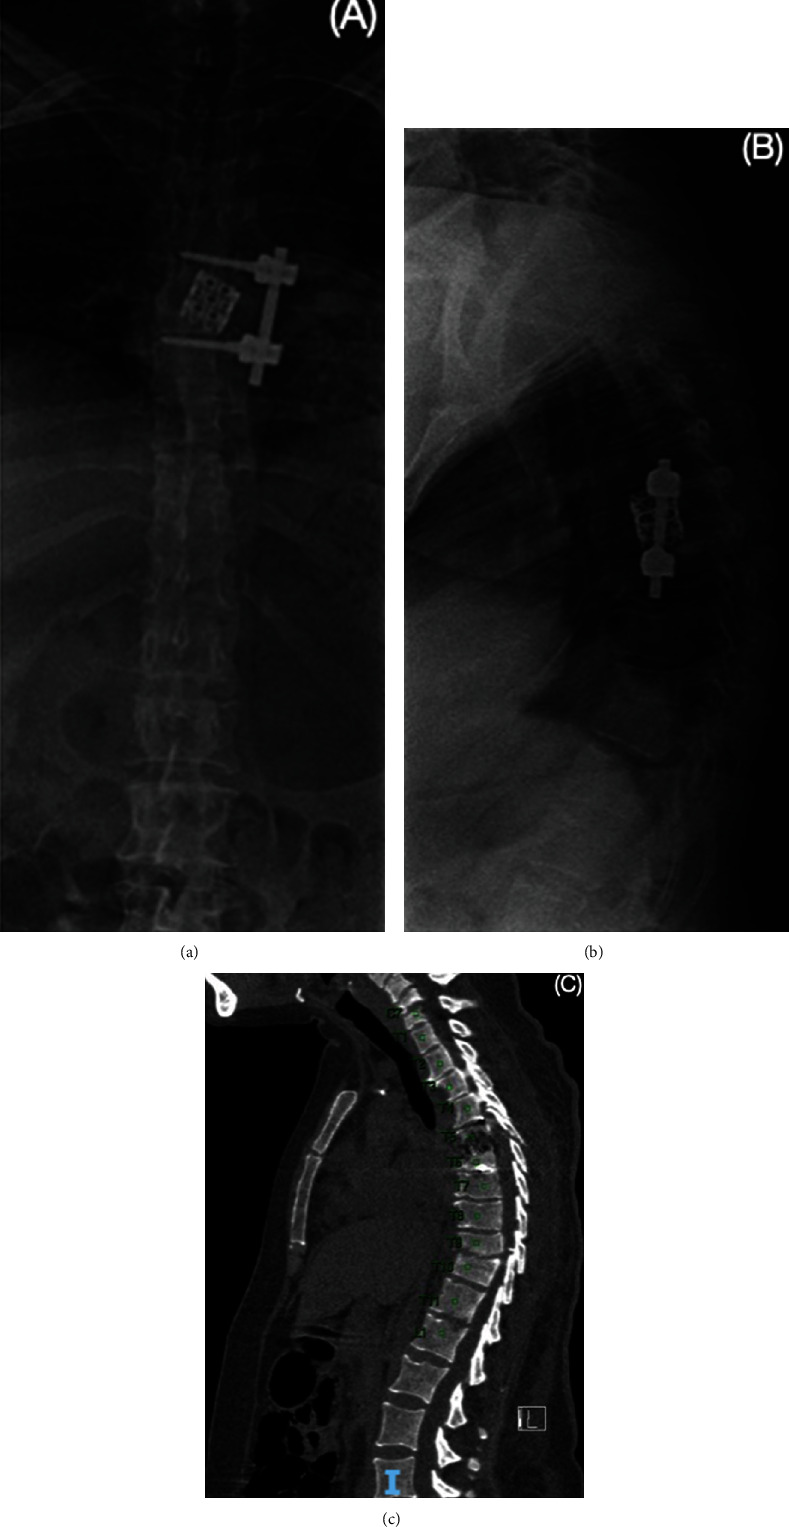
Postoperative x-ray of the thoracic spine: (a, b) postoperative changes are visualized at the level of the bony structures by left lateral instrumentation, with an intersomatic cage prosthesis at the level of T6-T7; (c) bone structures with postsurgical changes due to instrumentation in the thoracic spine, observing fixation screws and bar from the body of T4 to the body of T7.
